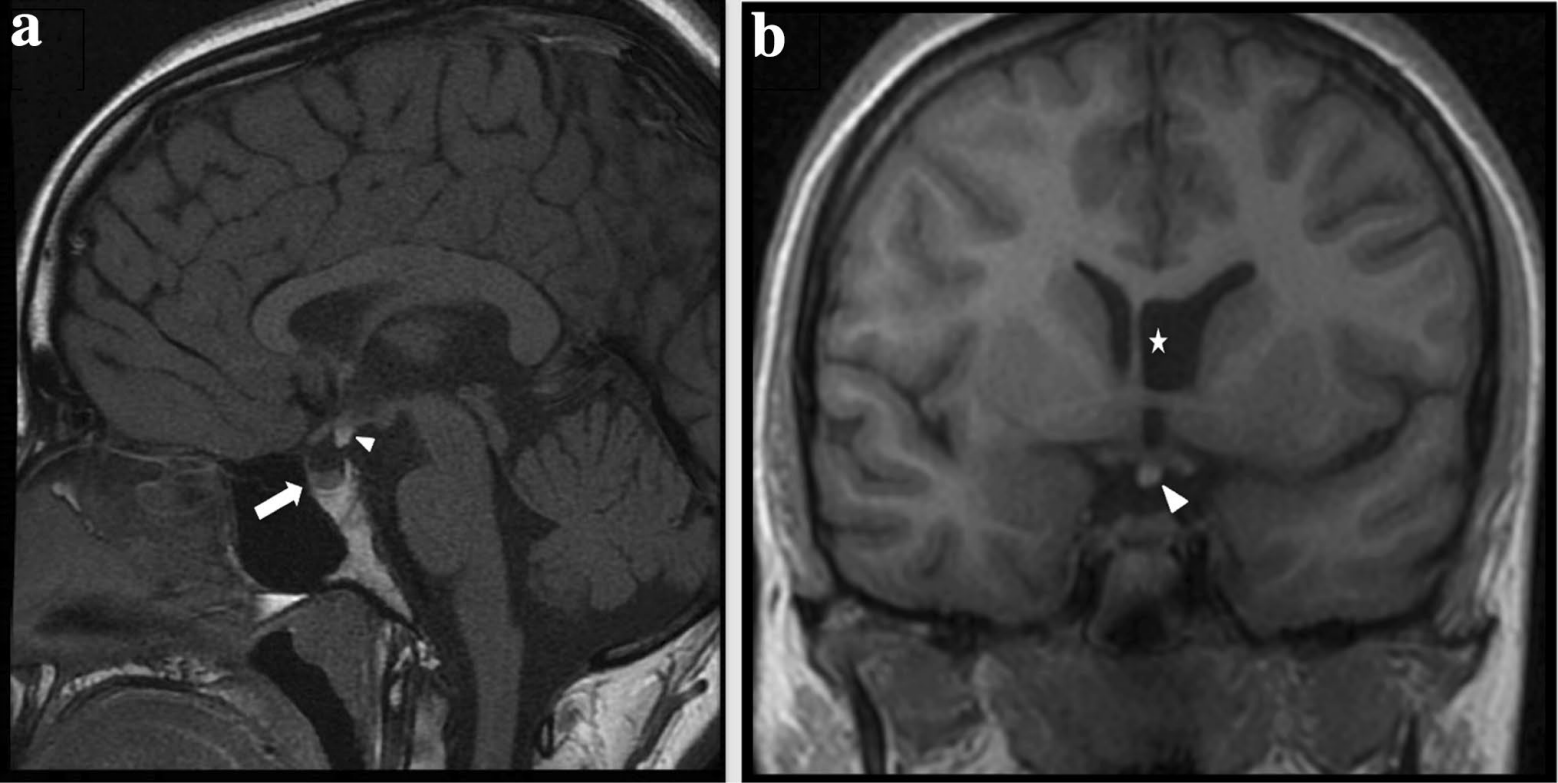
(ID 109): Ectopic posterior pituitary lobe*. a, b Unenhanced T1-weighted (T1w) midsagittal and coronal magnetic resonance images shows hyperintensity at the median eminence, which corresponds to ectopic posterior pituitary lobe (arrowheads). Anterior pituitary lobe appears normally localized although small and flattened (arrow). b Dysmorphic left lateral ventricles that appear fused with cyst of the cavum septum pellucidum (white star)
